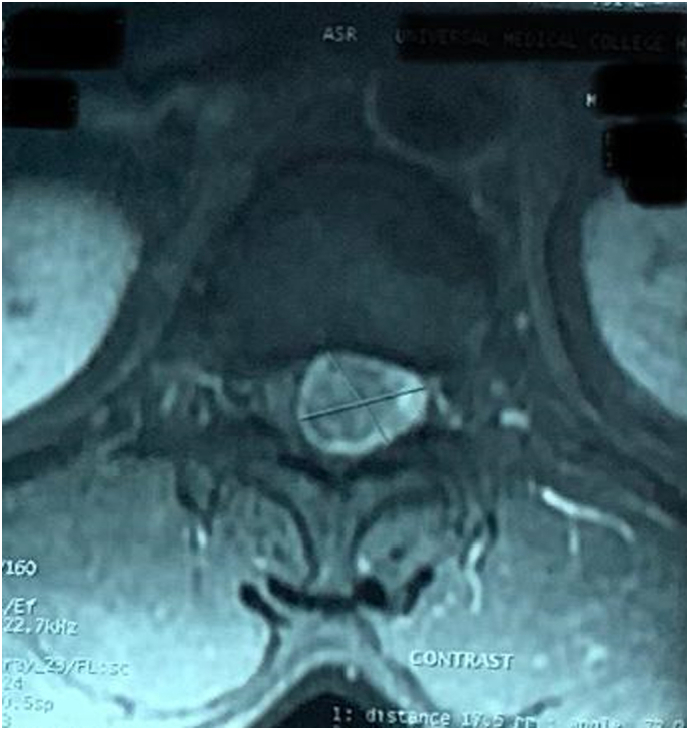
Contrast enhanced axial MRI showing the tumor.
